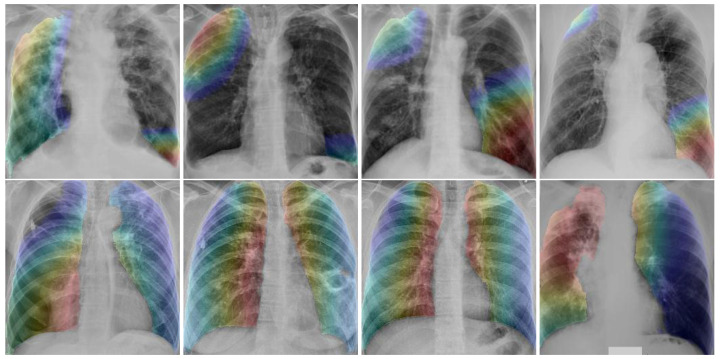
GradCAM visualizations on X-ray images. Top row shows examples of drug-sensitive TB and bottom row shows examples of drug-resistant TB.
